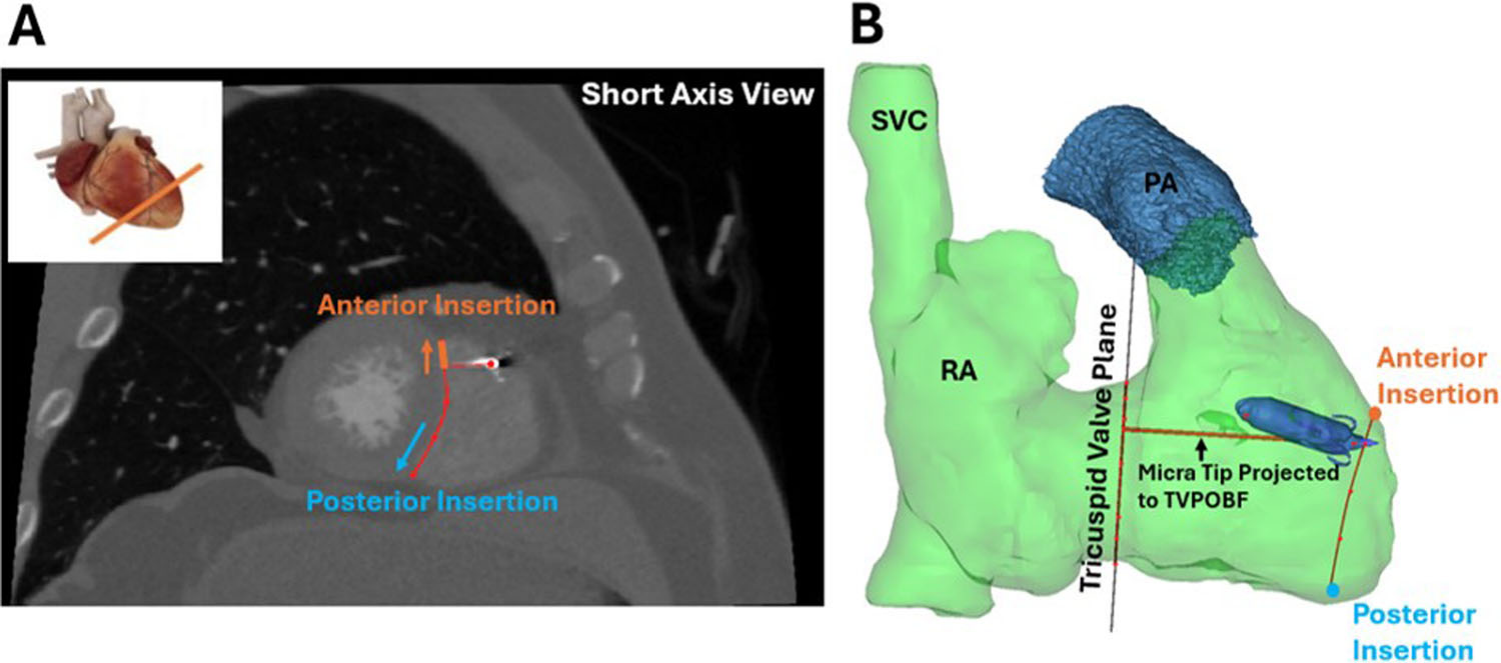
CT image processing to determine the tip location of Micra leadless pacemaker. (A): Short axis CT slice at the Micra tip location showing the distance from the projected Micra tip onto the septum to the anterior insertion (orange). (B): 3D representation of the right ventricle displaying the Micra tip distance onto the TVPOBF.
